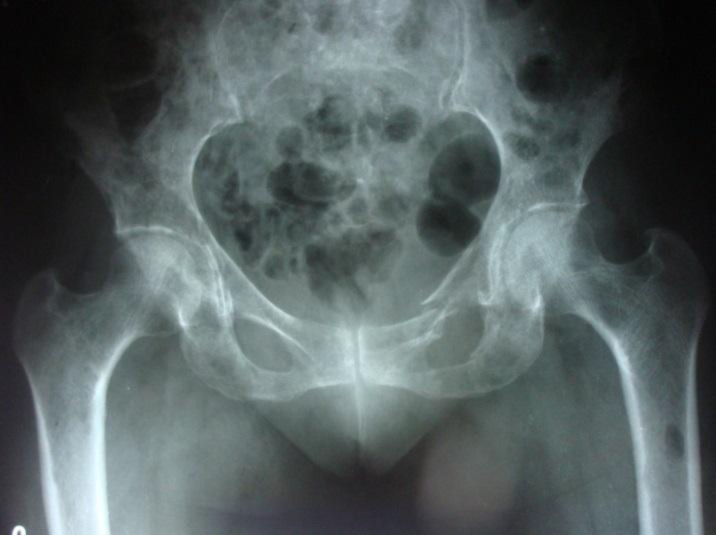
X-Ray Pelvis with both hips AP view- multiple lytic lesions in left subtrochanteric region.
